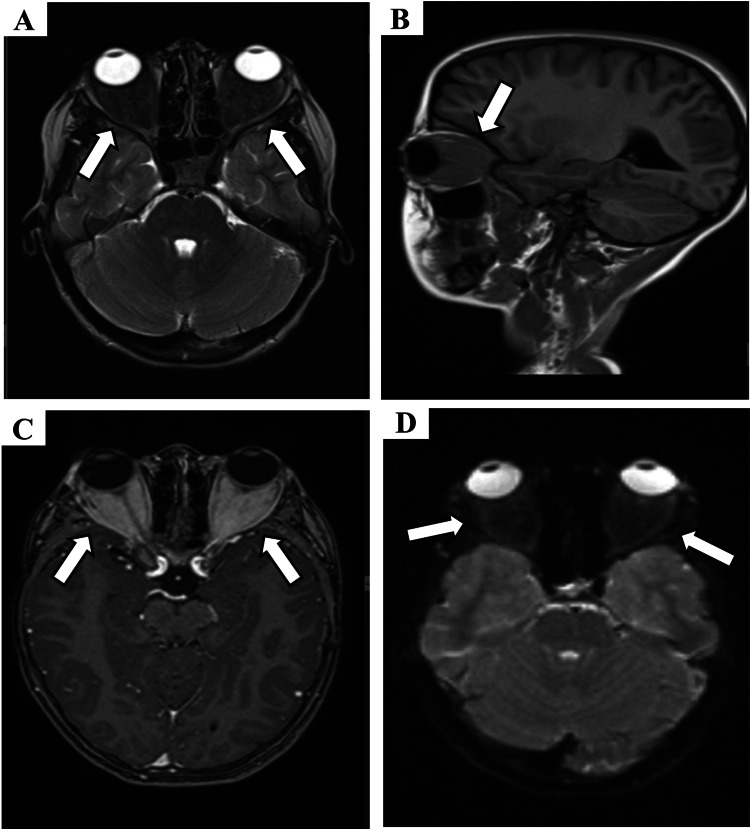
Orbital MRI characteristics Representative axial MRI sequences of the orbits. (A) T2-weighted image demonstrating bilateral, ill-defined intraconal infiltrates appearing hypointense. (B) T1-weighted image showing the infiltrates as isointense relative to extraocular muscles. (C) Post-contrast T1-weighted fat-suppressed image revealing homogeneous enhancement of the infiltrates. (D) DWI showing no restricted diffusion within the lesions. These findings are consistent with the diagnosis of idiopathic orbital inflammatory disease. MRI: magnetic resonance imaging; DWI: diffusion-weighted imaging
